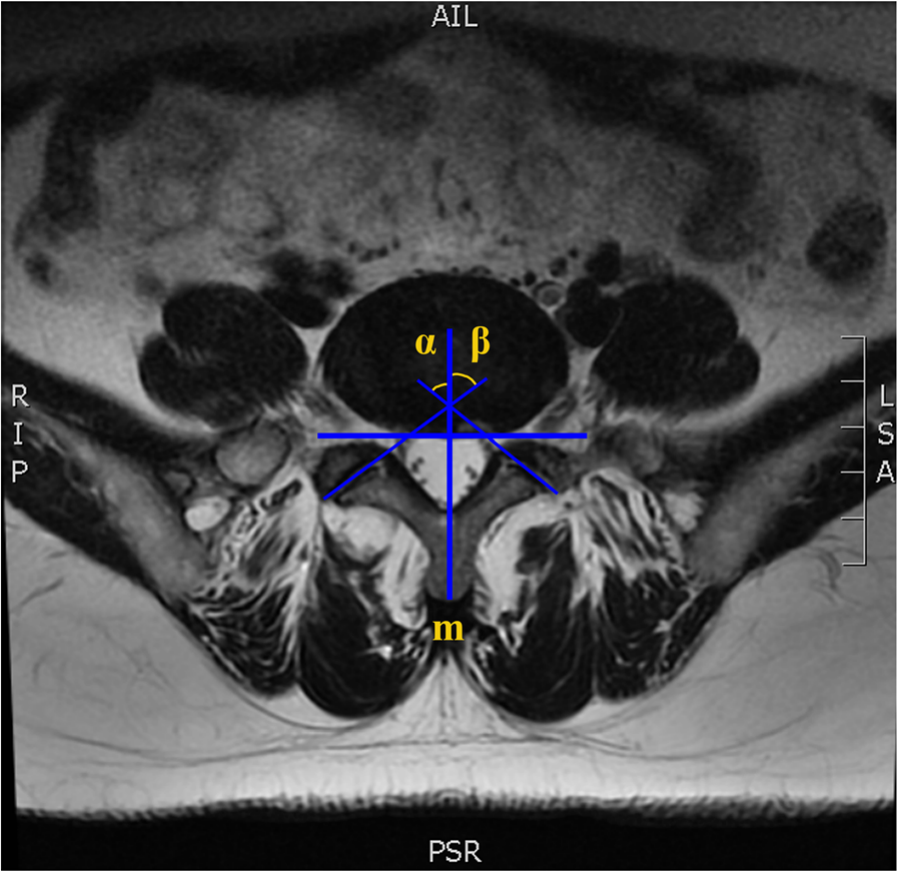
MRI measurement of facet joint orientation. m: middle line, a reference line bisecting the base of the spinous process; α: the angle between the middle line and the left facet line connecting the margins of the superior articular process;β: the right facet joint orientation
